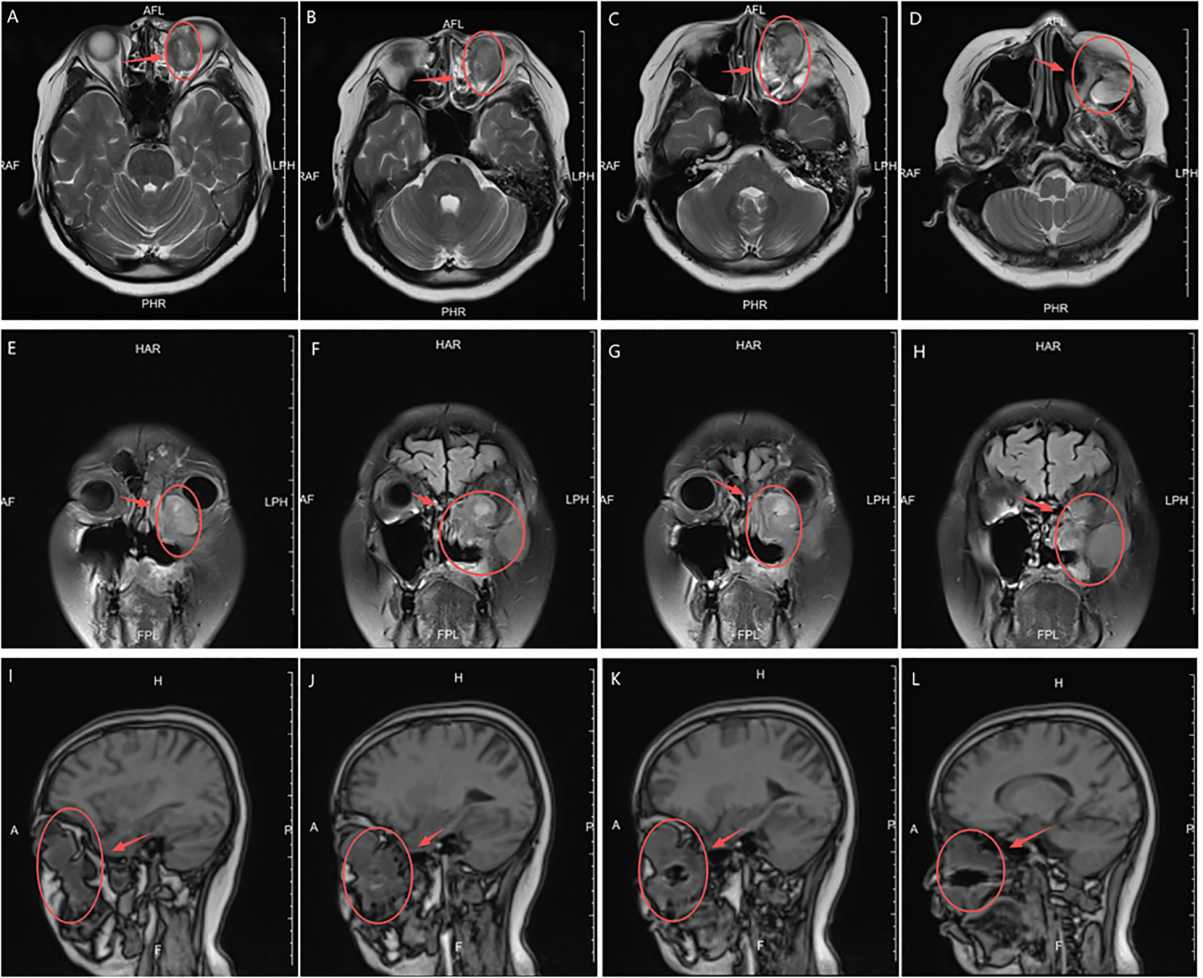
MRI Anatomical imaging: Findings consistent with (LGMFS) tumor recurrence involving the orbit, (as indicated by the arrows). (A–D) Axial T2-weighted image. (E–H) Coronal Short Tau Inversion Recovery (STIR) sequence image. (I–L) Sagittal T1-weighted image.
